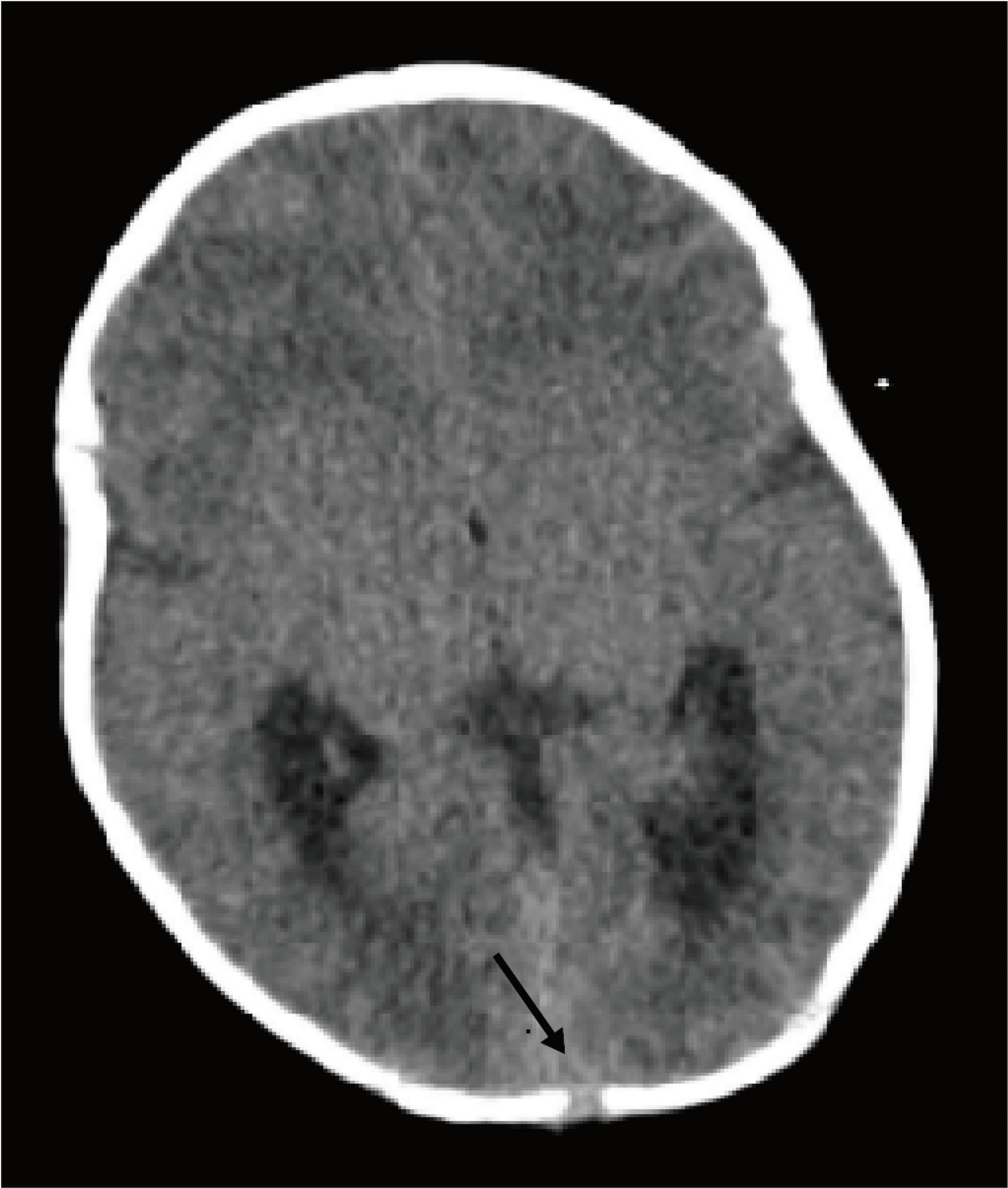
Axial computed tomography of the head revealed an encephalocele with intracranial communication.
